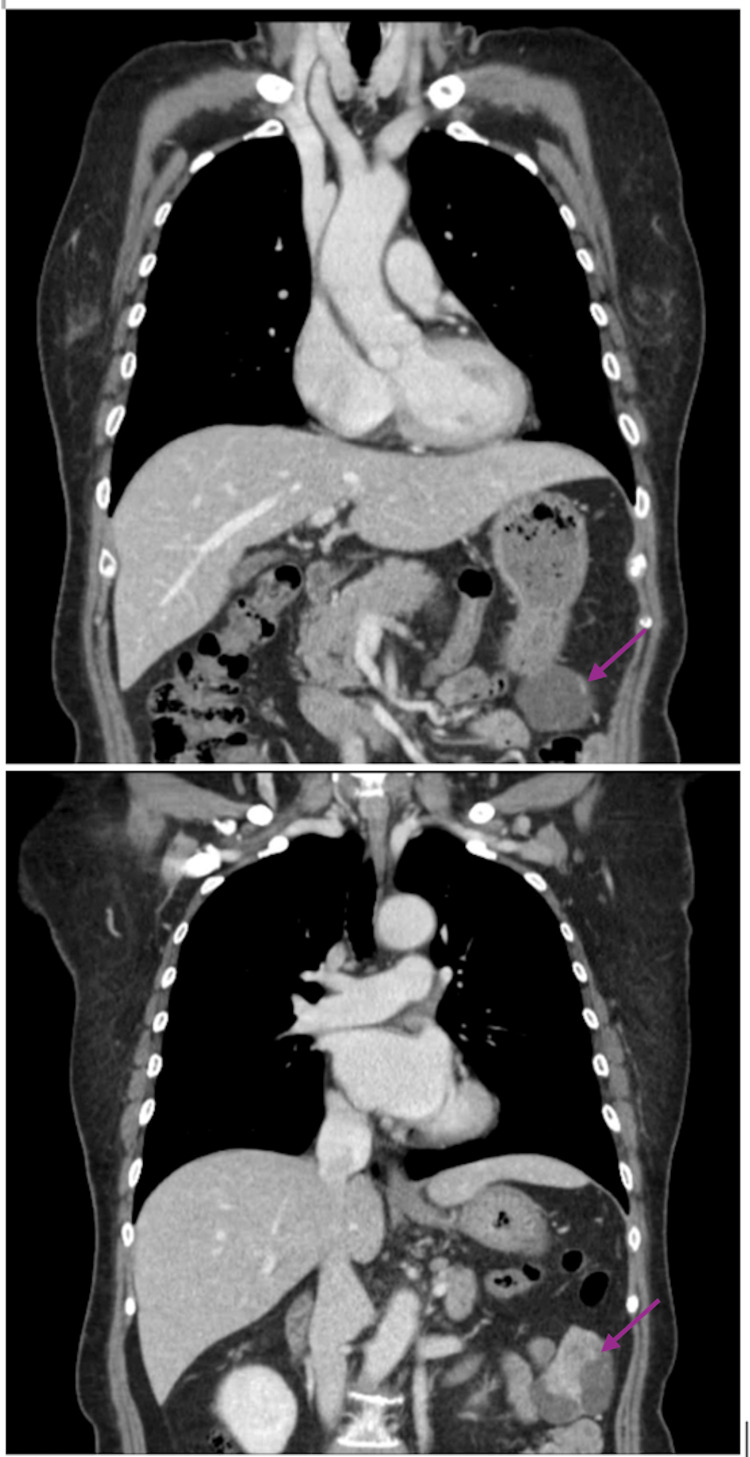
Coronal section of CT showing a mixed cystic-solid lesion (arrows) which appears to arise from the greater curve of the stomach.
